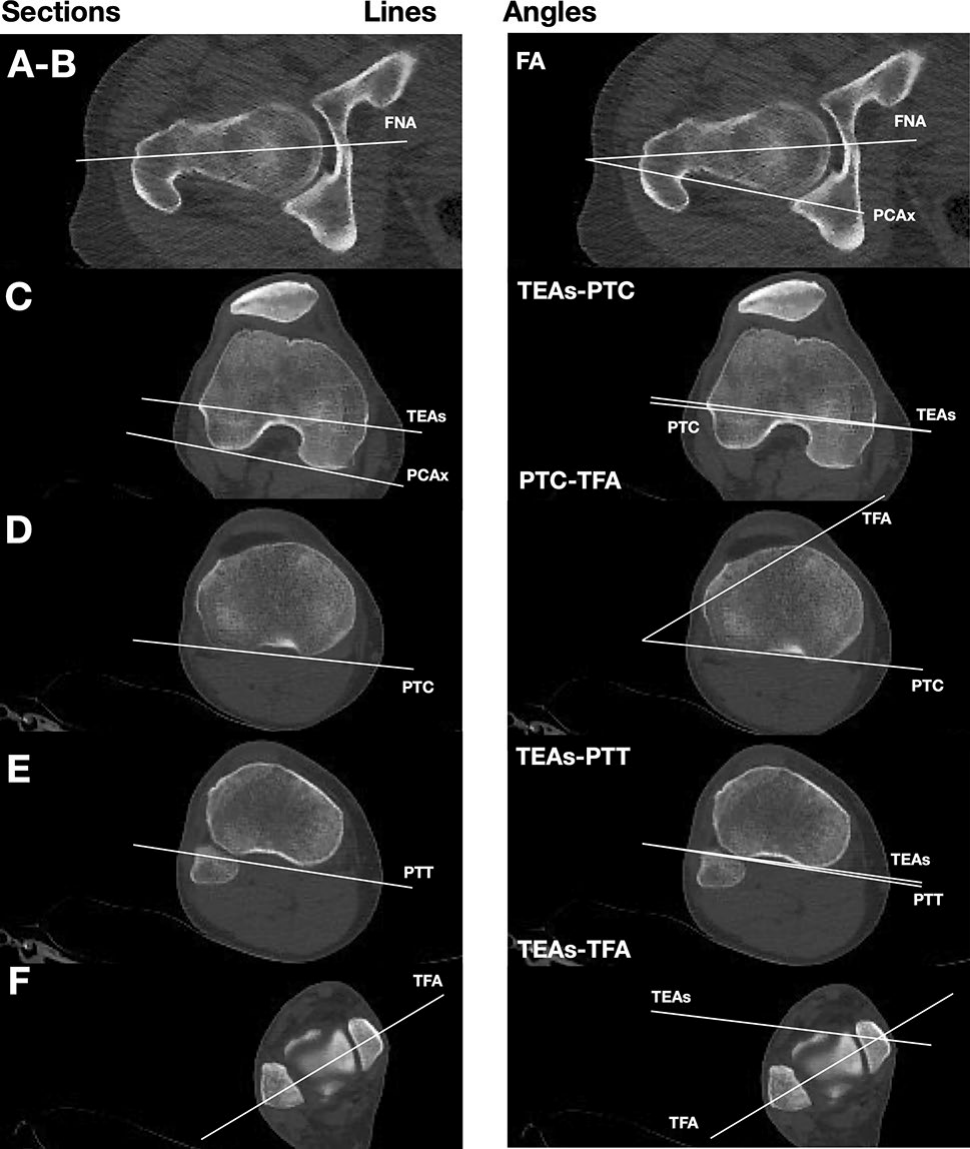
Torsion alignment of the lower limb: lines and angles analyzed in the present study. From the scout view, we find the following CT sections as explained in the text (a–f)
